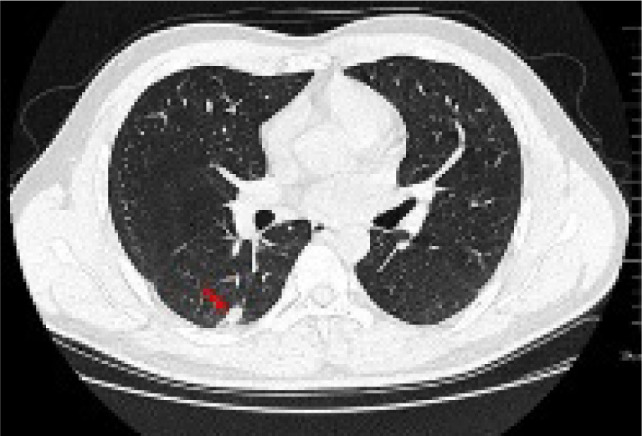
Lung CT shows that there is a calabash type density increase in the dorsal segment of the lower lobe of the right lung, the size of which was about 19×9 mm (red arrow).
